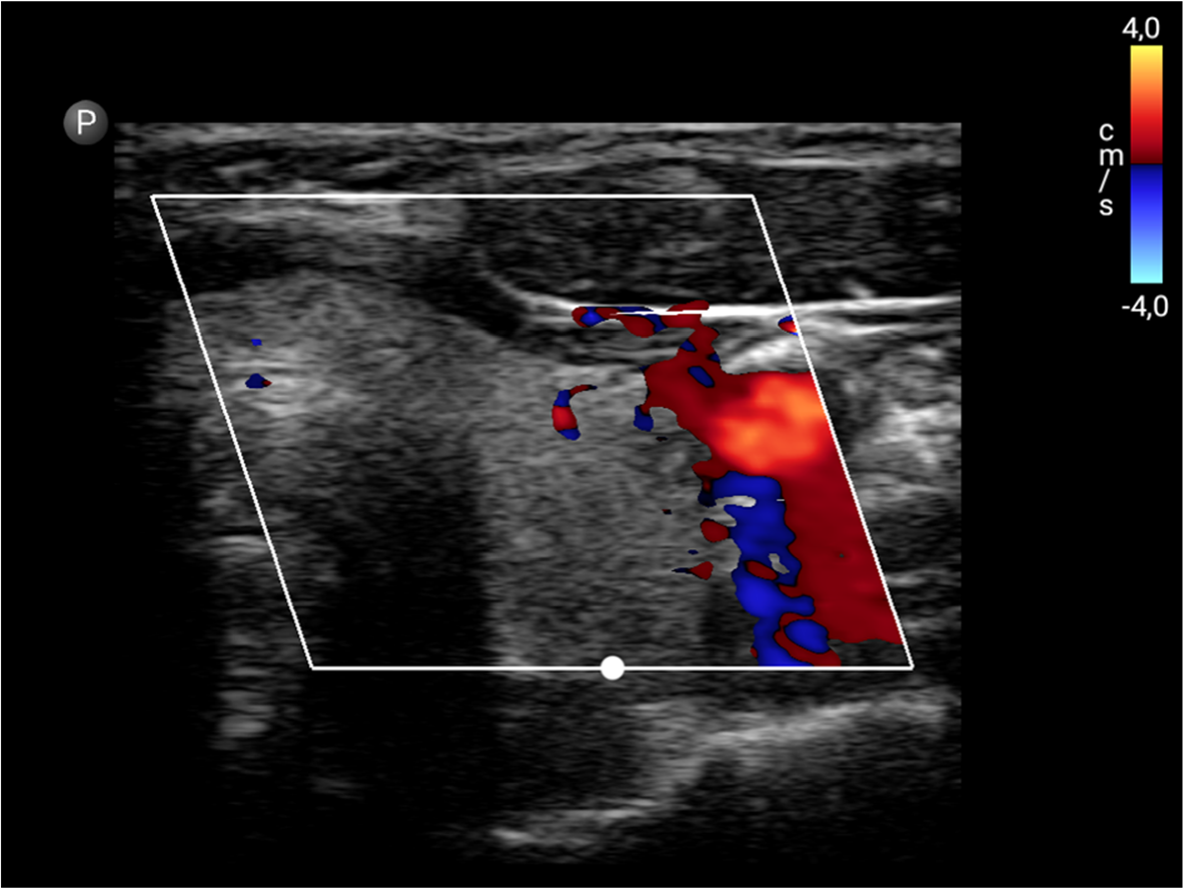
Philips Lumify linear transducer: Colour Doppler of the left thyroid lobe including the carotid artery
